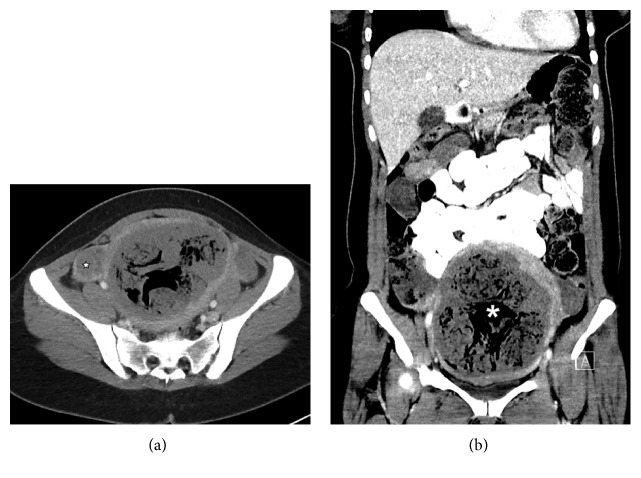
Pelvic CT performed on postprocedure day #26. Axial image (a), at the level of the ovaries (star), and (b) coronal image of the uterus show a large amount of intrauterine air (asterisk), including nonlinear, extravascular air, raising the suspicion of pyomyoma in the proper clinical setting. Patient also had unexpected small bilateral pleural effusions and moderately sized pericardial effusion (not shown).
